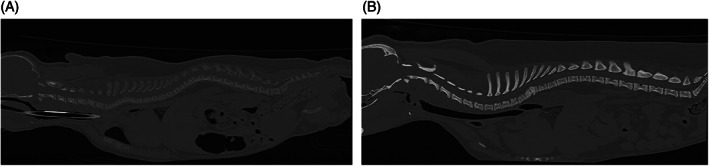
(A) Computed tomography images of the entire spine of Pug 1.1♀ with VDDR type 1A showing generalized decrease in bone density (attenuation). Endplate widening is also noted. (B) Computed tomography images of the entire spine of an age‐matched control pug.
